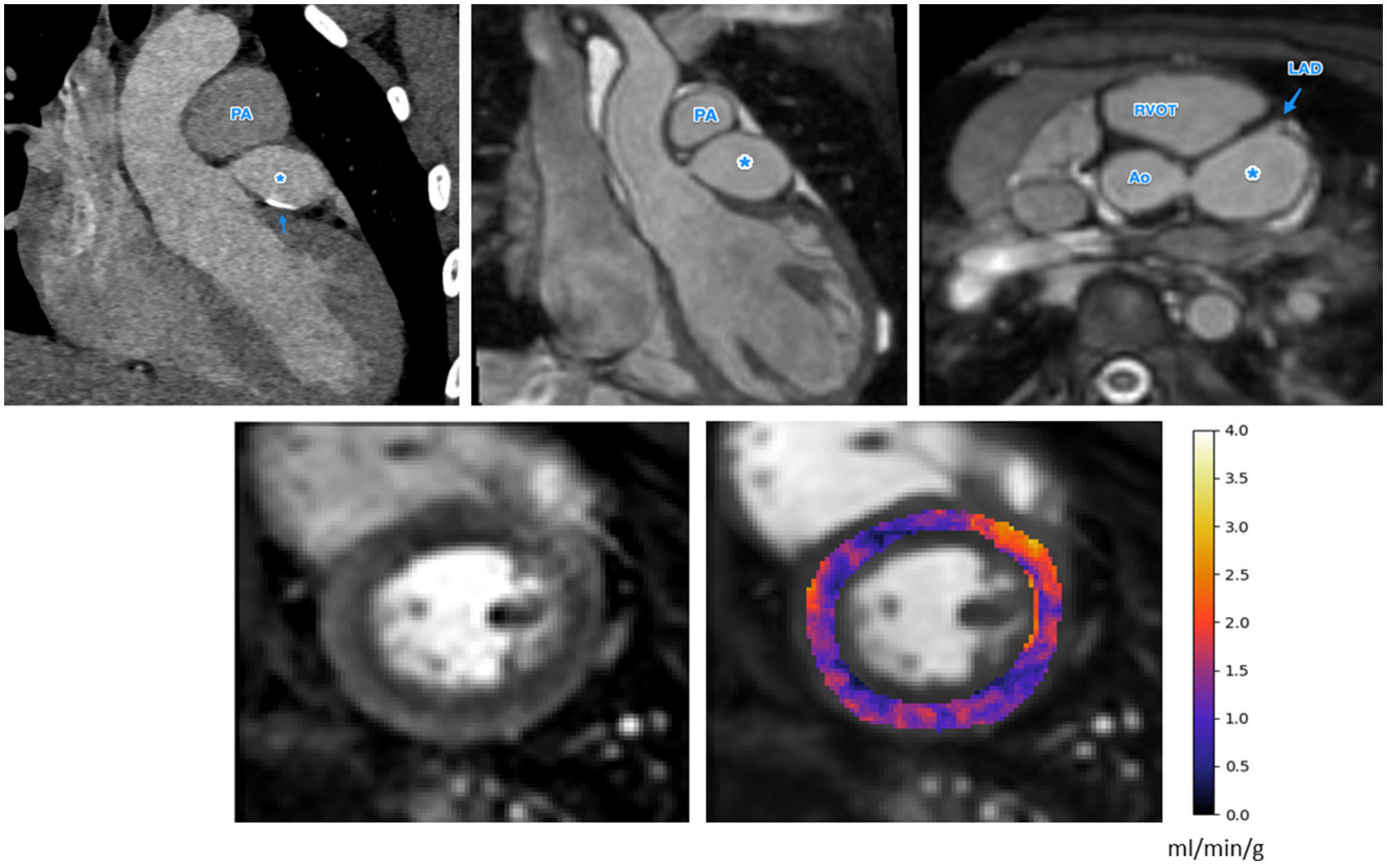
The anatomical images (top row, CT left, MR center and right) and stress perfusion CMR with quantitative MBF map (bottom row) for a patient with Kawasaki disease and a giant aneurysm of the LMCA, indicated as *in the anatomical images. There is also calcification of the LMCA seen on the CT image (top left), indicated by the arrow. There is globally low MBF values, possibly indicating microvascular dysfunction (bottom right). Ao, Aorta; PA, pulmonary artery; MPA, main pulmonary artery; RVOT, right ventricular outflow tract; LAD, left anterior descending artery.
